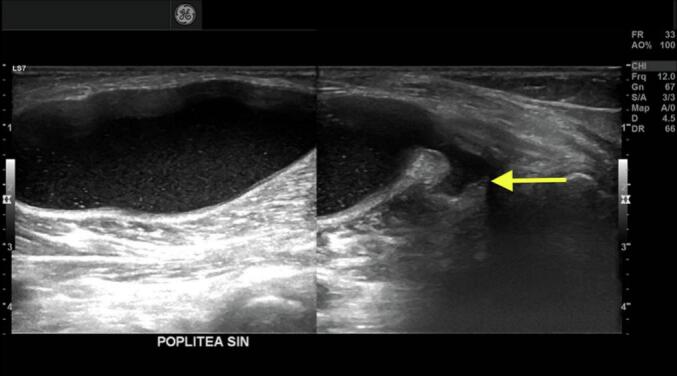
The ultrasonogram of the popliteal mass confirmed a cyst.
